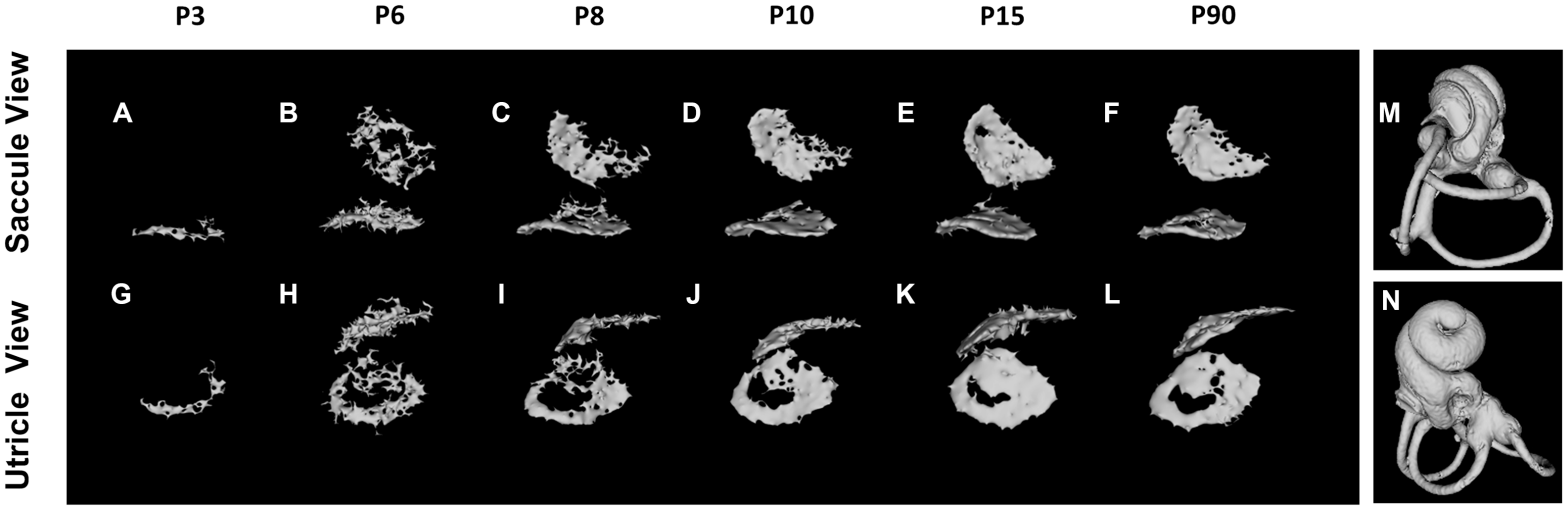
Otoconium mineralization. The utricular otoconia are first detected at P3 by reconstructed micro-computed tomography (A,G), whereas the saccular otoconia are identified at P6 (B,H). The otoconium mineralization gradually develops between P6 and P10, and the calcium depositions on the otoconia on the utricle and saccule are almost completed at P15 (E,K), the computed tomography images of which are almost identical to those at P3–4 Mo (F,L). The orientations of Saccule and Utricle view are designated as (M,N).
